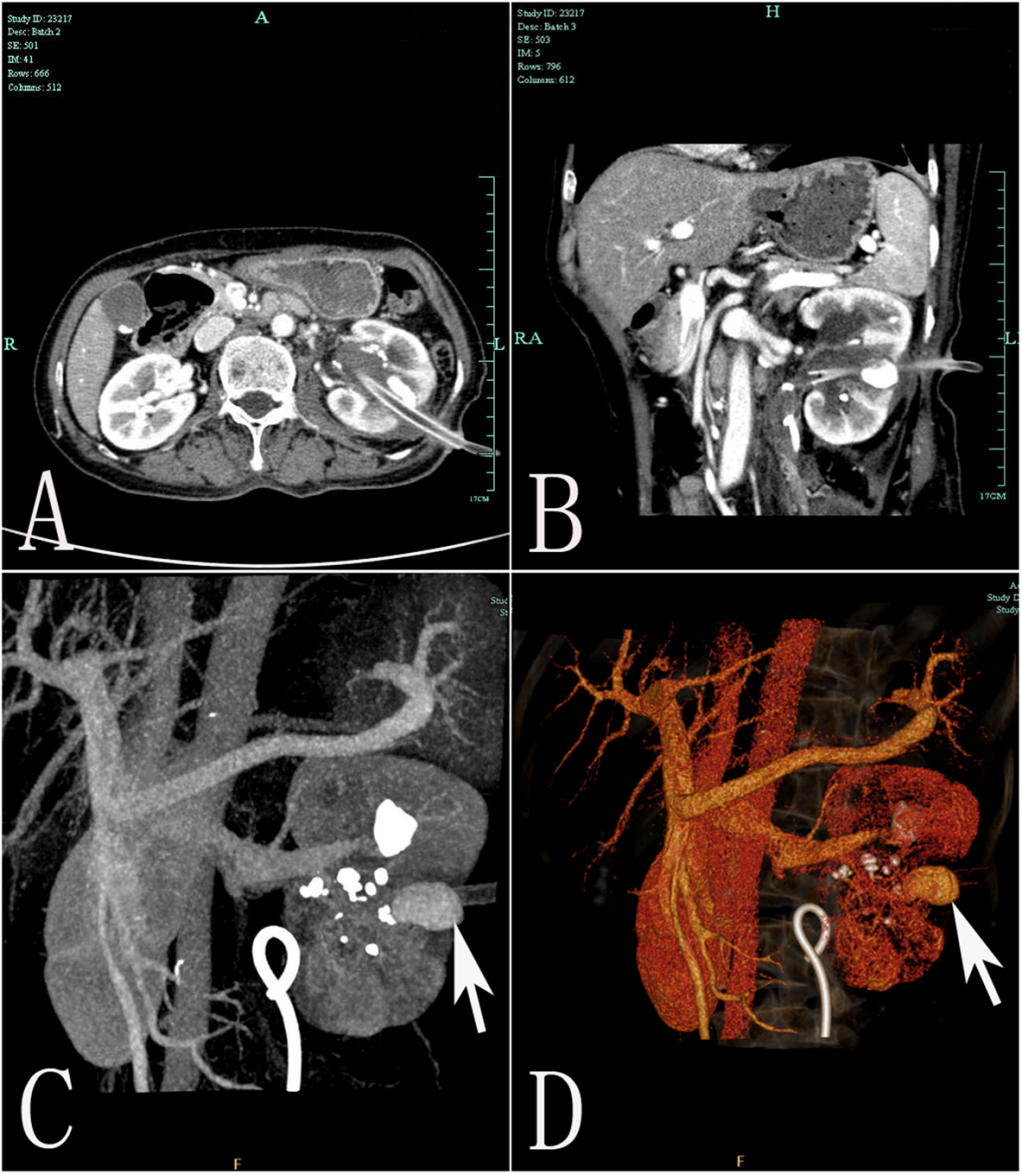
Imaging of CT. A) Axial contrast enhanced CT demonstrates the pseudoaneurysm (an oval structure) arising from the lower pole of the left kidney, the pseudoaneurysm was located near the percutaneous nephrostomy tract. B-D) Coronal reformatted (B), maximum intensity projection (C) and volume rendering (D) images of CT angiography reveal the pseudoaneurysm (arrow) arising from the lower polar segmental artery.
